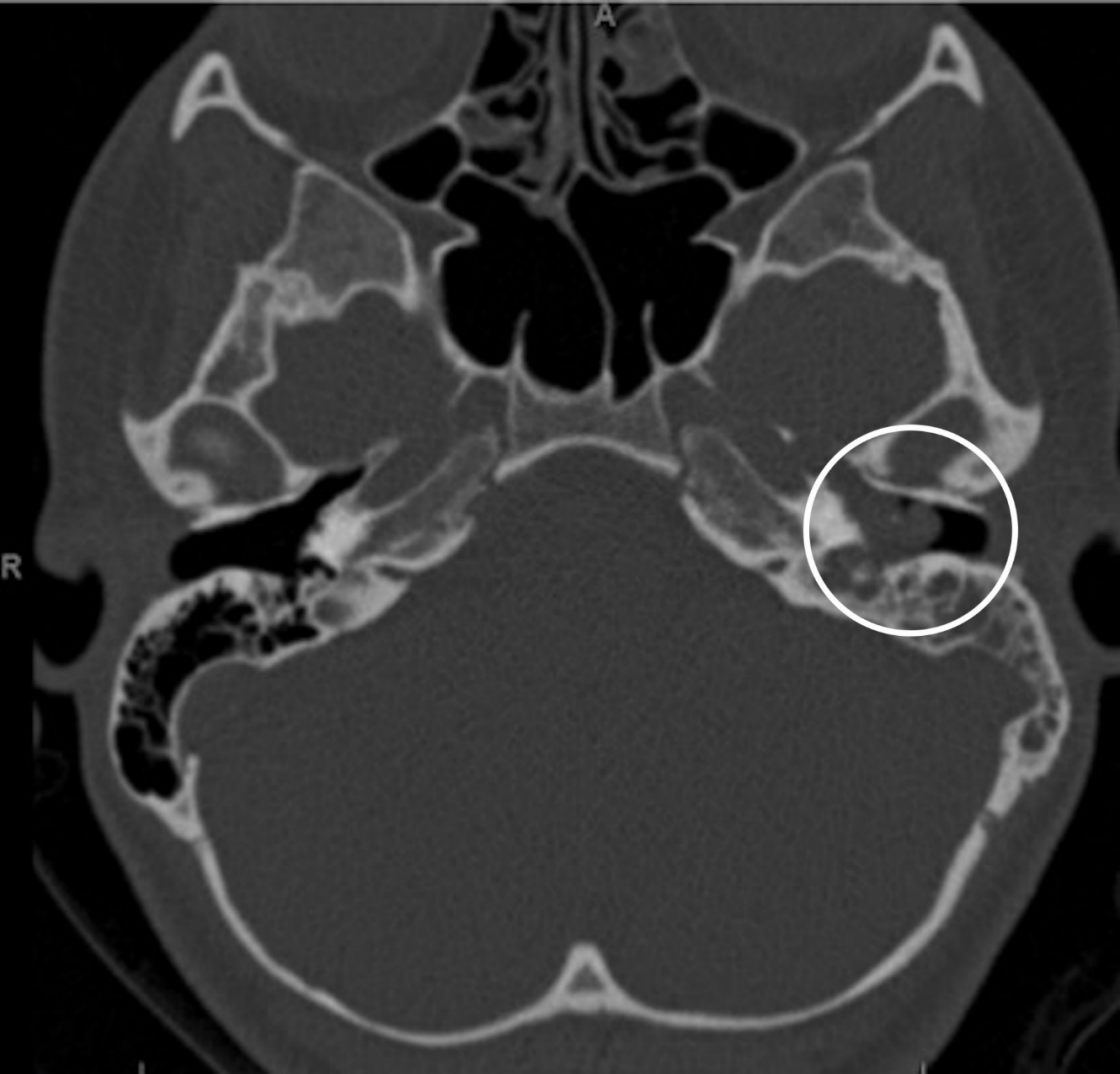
Figure 2 Axial-cut CT Showing Left Middle Ear Mass
